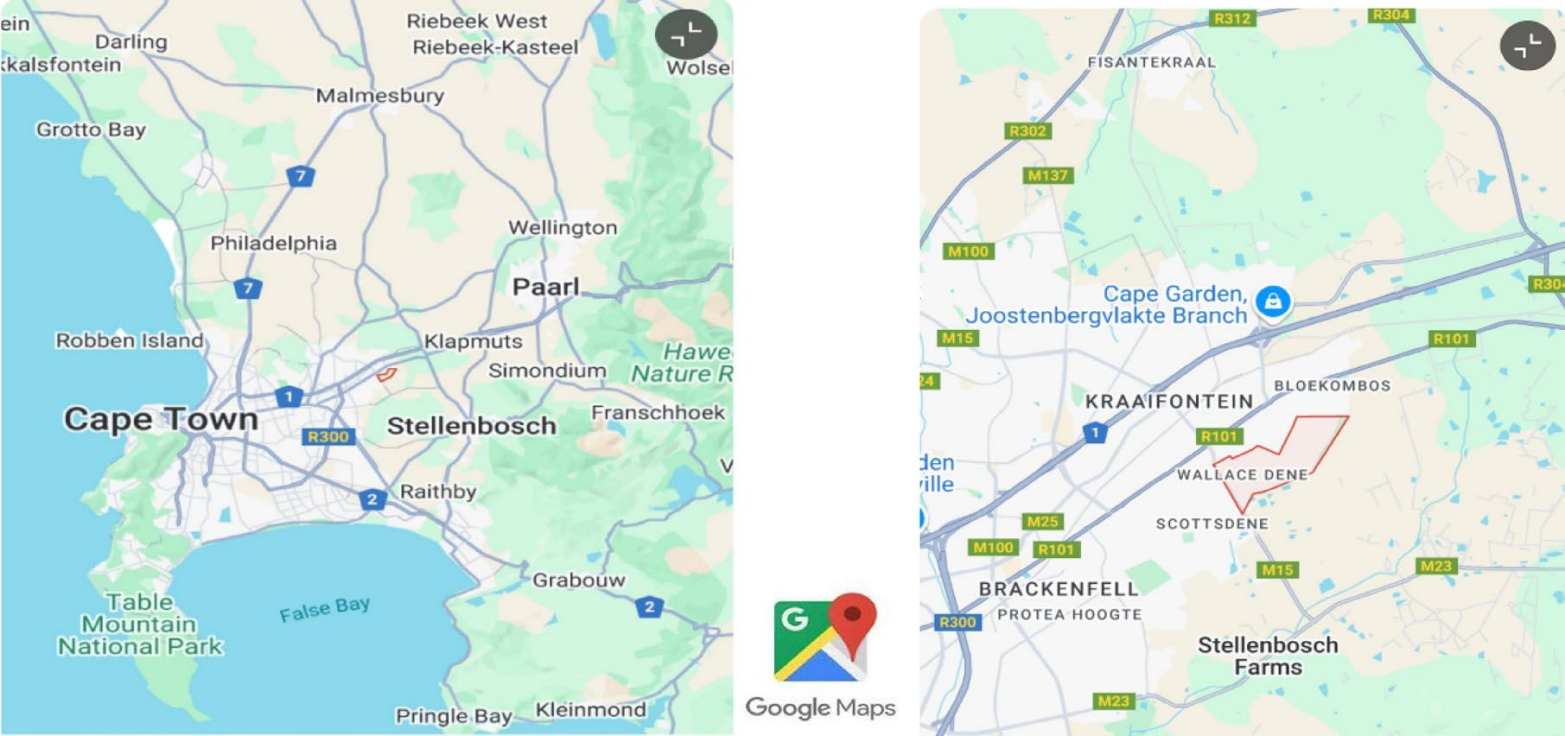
Geographical location of Wallacedene in Kraaifontein, CT. Zoom close-up level map. Source: Google Maps (2021)
